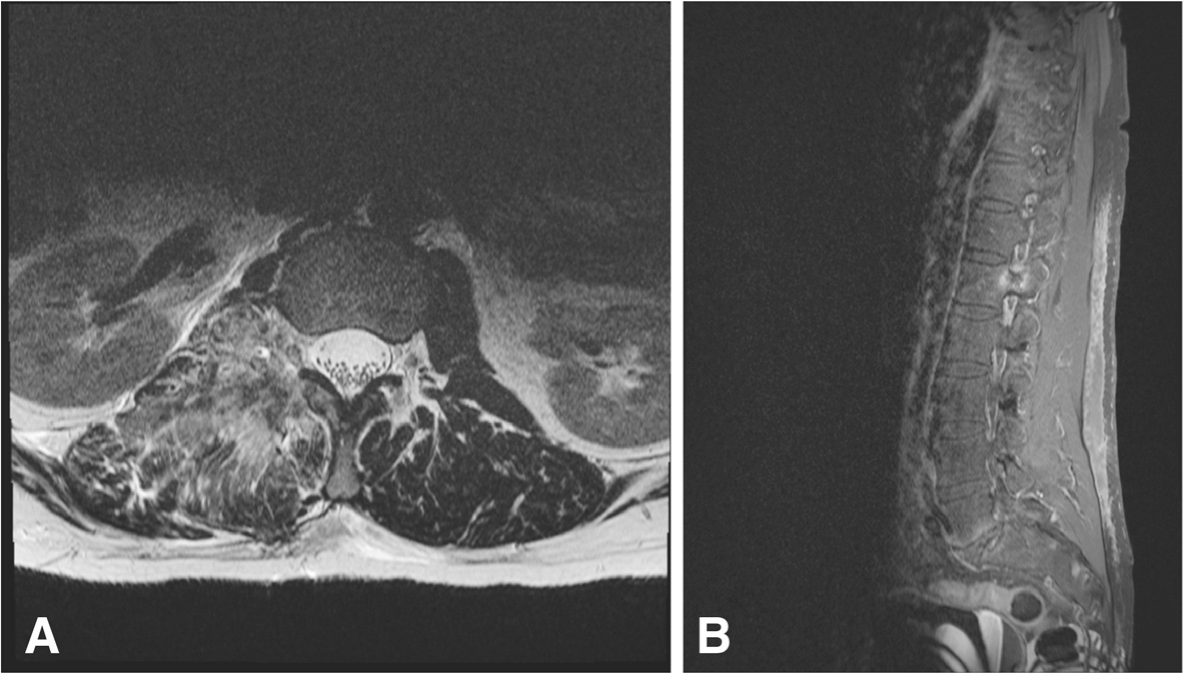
Postoperative T2-weighted, contrast-enhanced MRI confirmed complete tumour removal in axial (A) and sagittal (B) planes.
